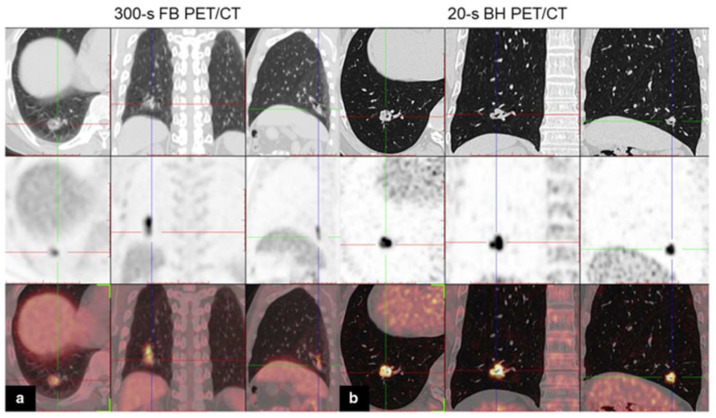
[^18^F]fluorodeoxyglucose (FDG) PET/CT images of a 59-year-old man with lung adenocarcinoma. Axial, coronal, and sagittal chest CT (top row), PET (middle row), and PET/CT (bottom row) images of a solitary solid lung nodule in the right lower lobe: (a) FB PET/CT; (b) BH PET/CT. Notice how the uptake area appears blurred owing to breathing artifacts in the FB PET image. Image (partially) taken from Cheng et al. CC BY 4.0 License: http://creativecommons.org/licenses/by/4.0/ [25].
